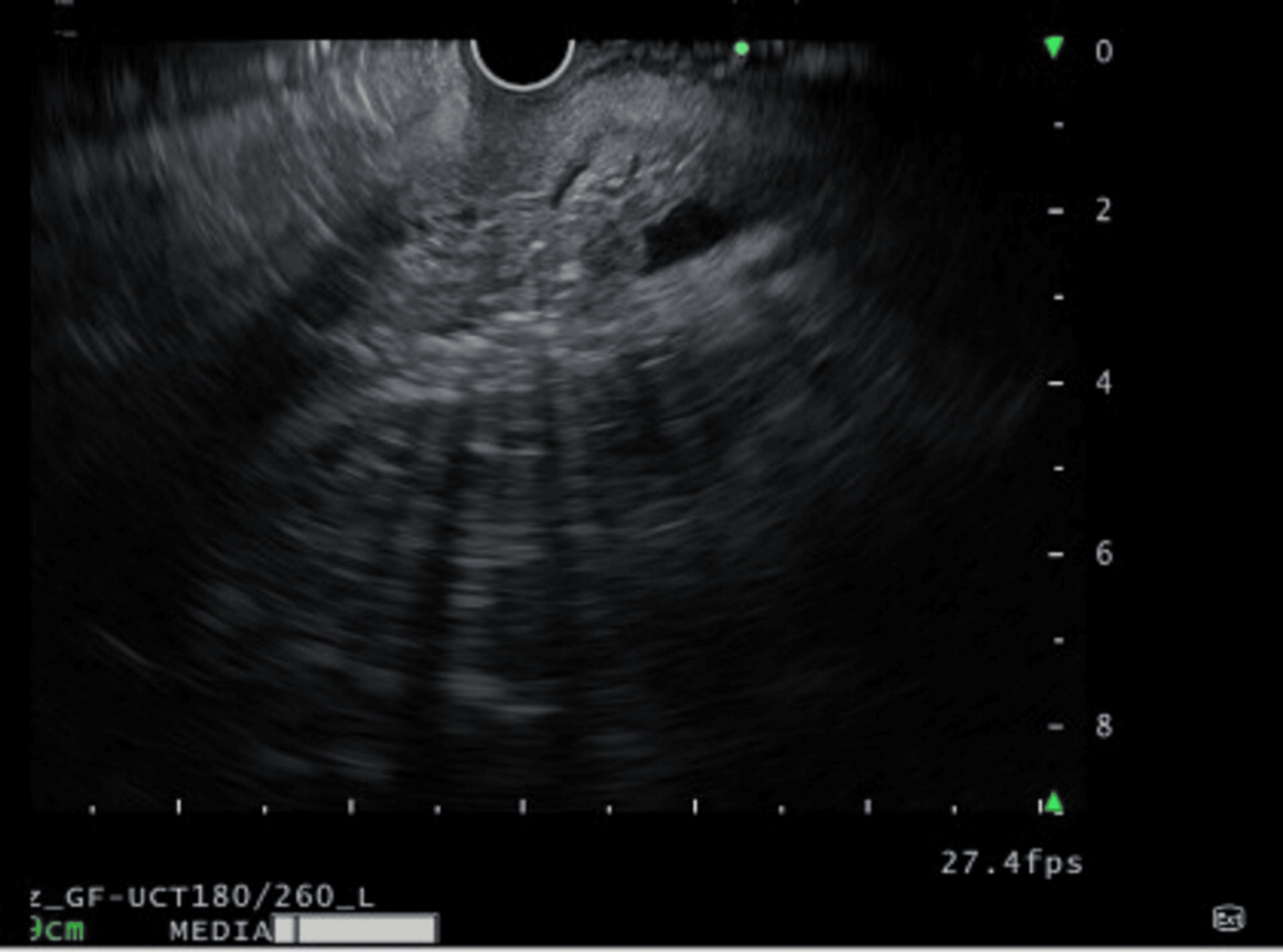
EUS showing normal pancreas with homogeneous echotexture without any focal masses. EUS: endoscopic ultrasound
